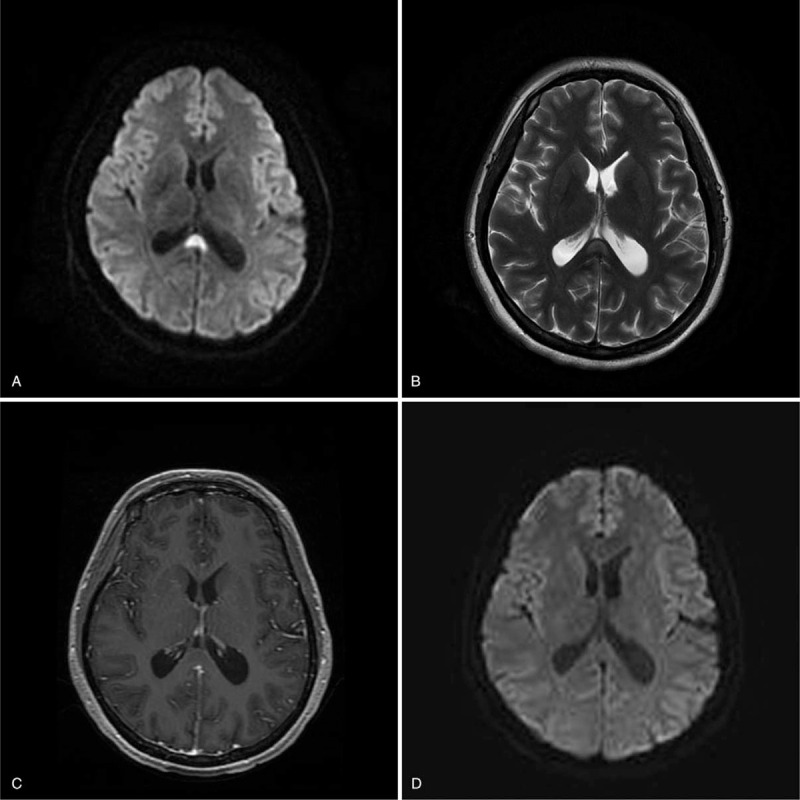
In case 1, a 26-year-old woman presented with fever and headache. The initial enhanced magnetic resonance 3.0 T MRI indicated a region of the splenium hyperintensities on the DWI image (A) and FLAIR (B), without contrast enhancement effect (C). The follow-up 1.5 T MRI at 26 days later indicated the complete disappearance of the splenial lesion (D).
